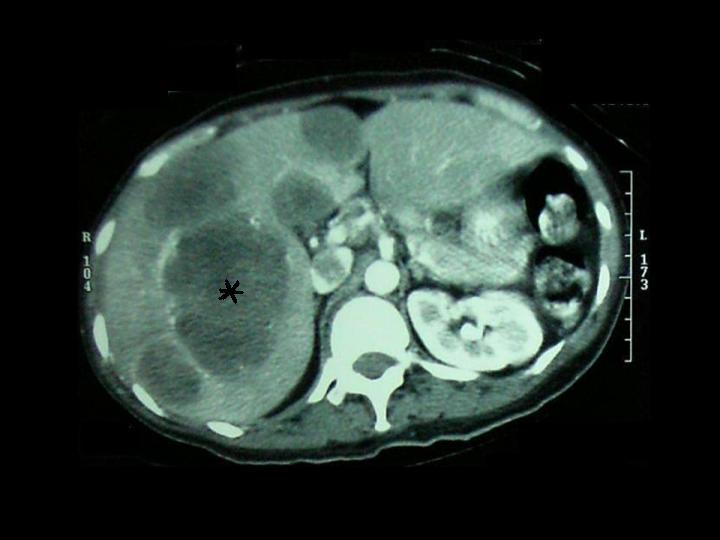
An axial contrast-enhanced CT scan of the liver demonstrating multiple liver metastases of varying sizes (the largest marked with an asterisk).
